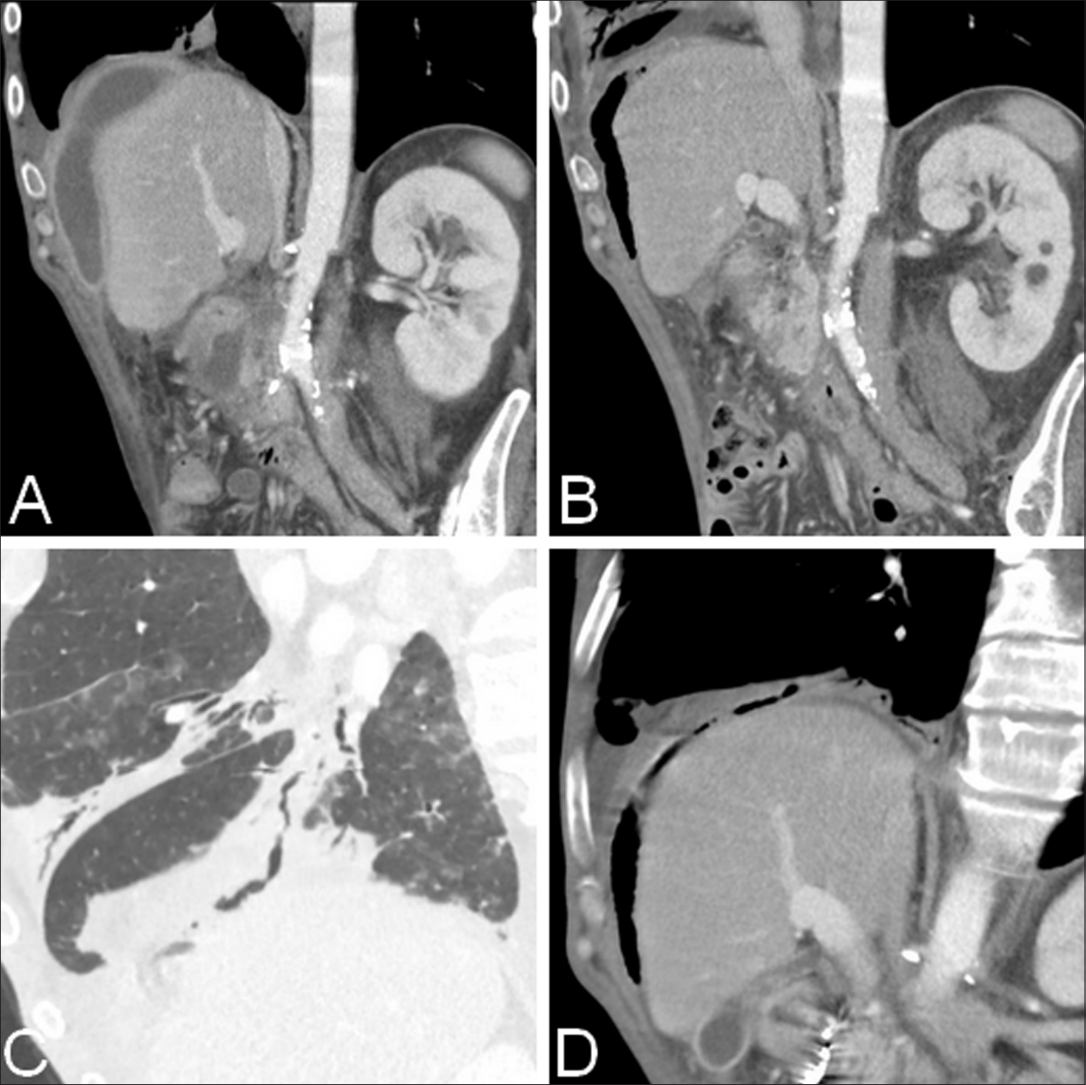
(A) CT showing a pseudocyst underneath the right portion of diaphragm, above the liver. (B) The last exam reveals a significant decrease and apparition of gas in the pseudocyst, suggestive of spontaneous fistula. (C, D) Coronal oblique MPR views respectively in the pulmonary and abdominal windows showing a distal bronchi communicating by a fistulous way to the pseudocyst through the diaphragm.
